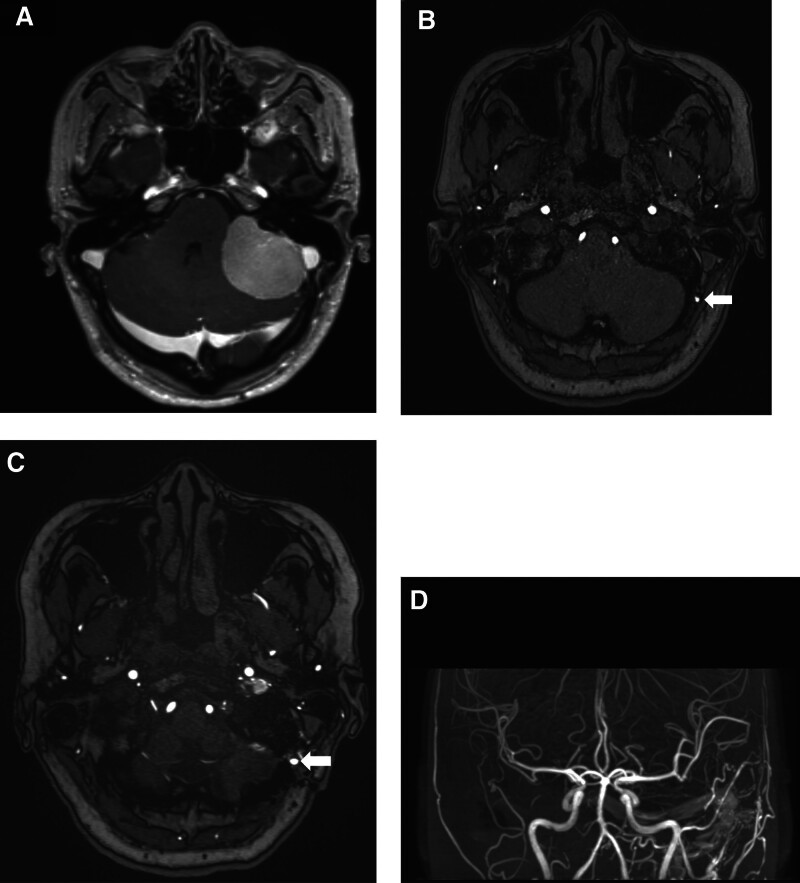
(A) Preoperative magnetic resonance imaging showing 4-cm-sized cerebellopontine angel meningioma. (B) Preoperative noncontrast-enhanced time-of-flight–magnetic resonance imaging showing a normal occipital artery (arrow). (C) Postoperative noncontrast-enhanced time-of-flight–magnetic resonance imaging showing a dilated occipital artery (arrow). (D) Postoperative magnetic resonance angiography showing arterialized transverse and sigmoid sinuses.
